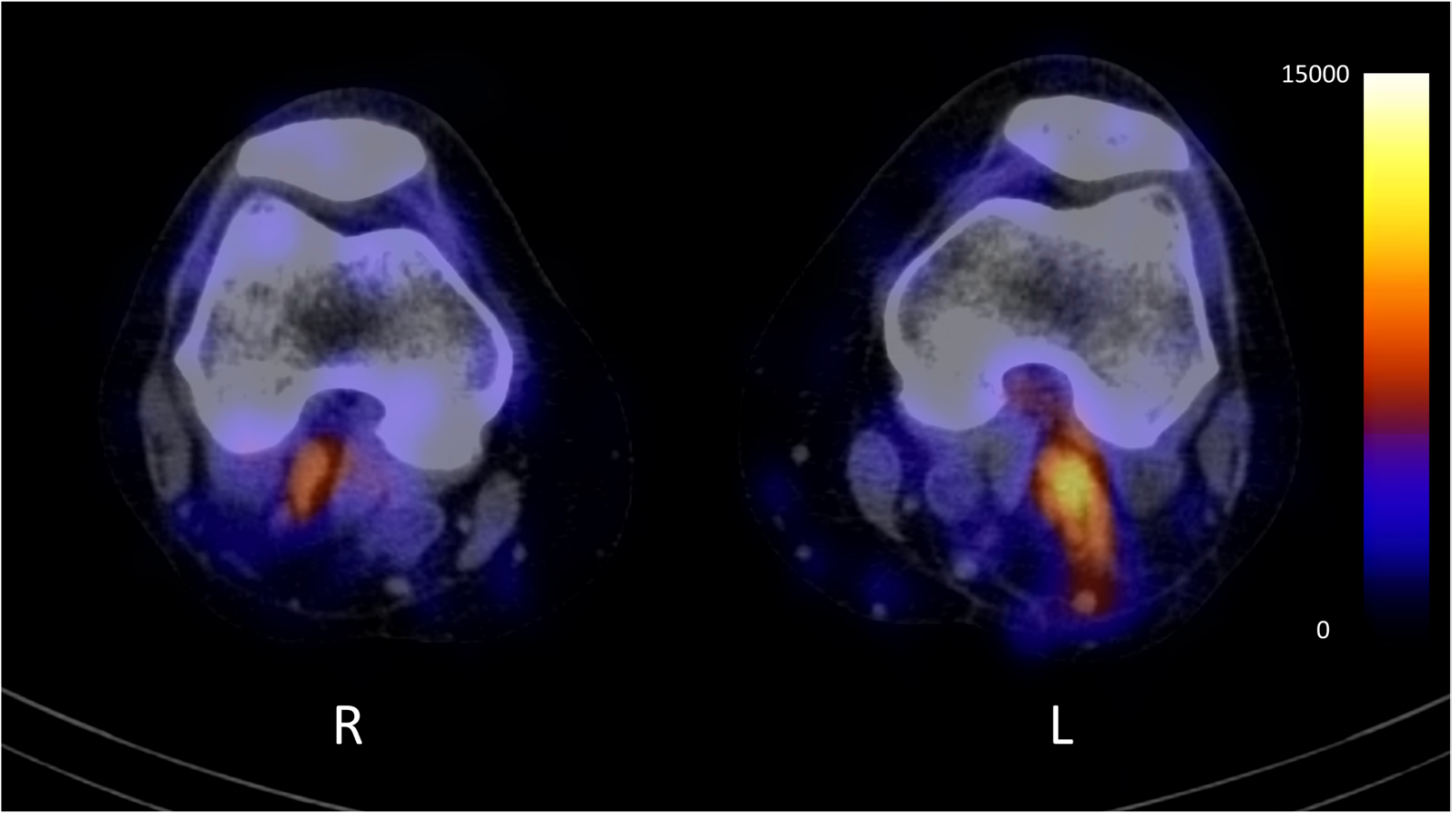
Focal uptake of ^99m^Tc-fucoidan in deep vein thrombosis of the left popliteal vein ^99m^Tc-fucoidan SPECT/CT image of a 50-year-old woman recently diagnosed with a deep vein thrombosis of the left popliteal vein. Focal uptake can be observed at the thrombus site. The scale bar is measured in absolute counts
